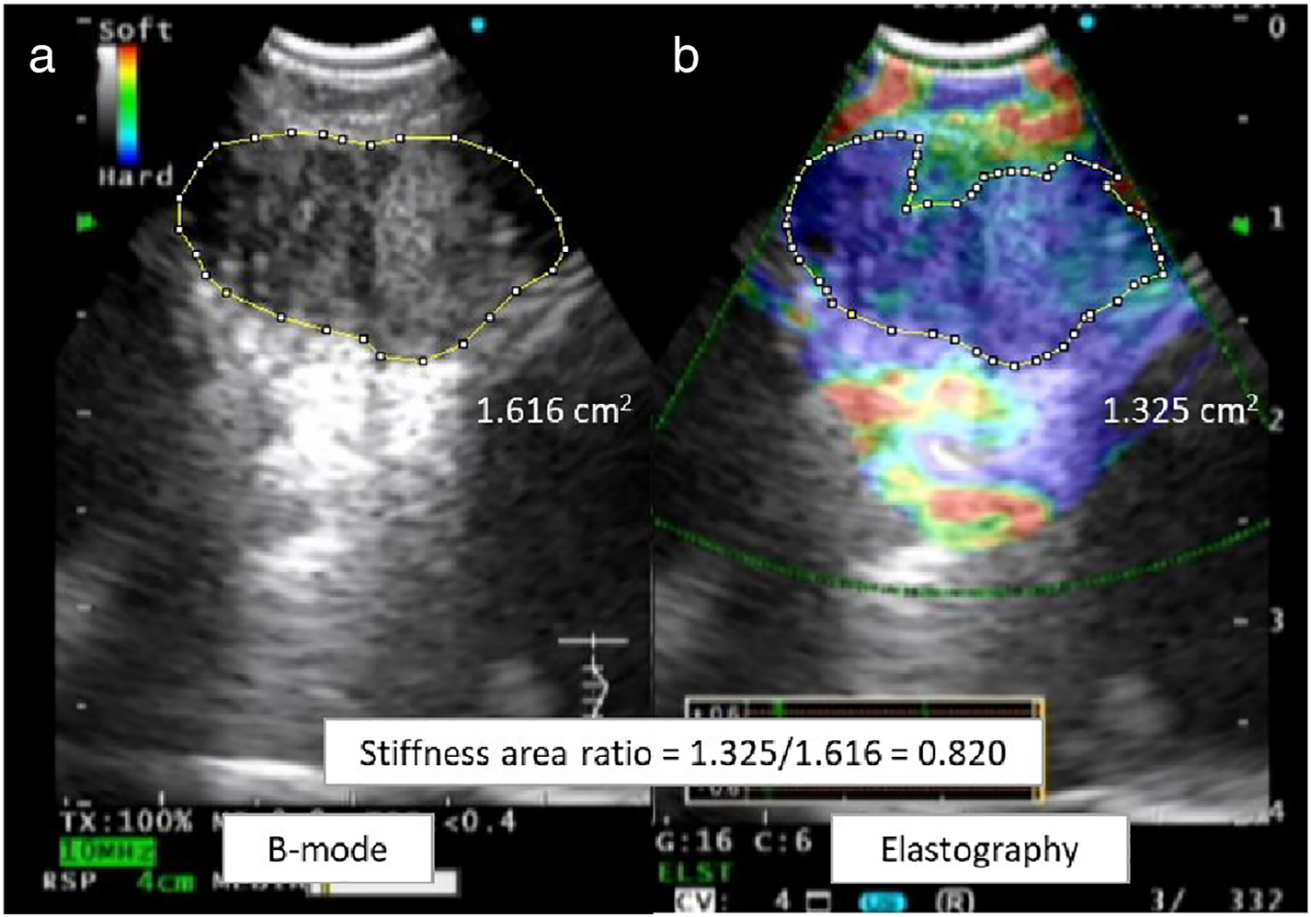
Quantitative evaluation of endobronchial ultrasound (EBUS) elastography using ImageJ. The stiffness area ratio was calculated by dividing the blue area (indicating stiffness) by the entire area of the lymph node. (a) The entire area of the target lymph node was manually enclosed and measured on the B‐mode sonographic image. (b) The stiffness area within the lymph node was manually enclosed and measured on the EBUS elastography image. The stiffness area ratio was computed after EBUS‐transbronchial needle aspiration.
